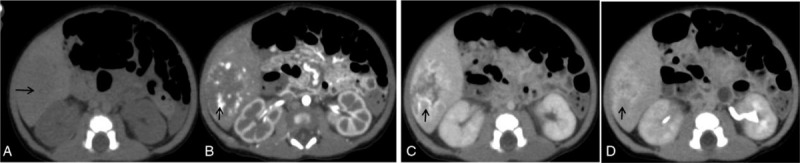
A 2-day-old female neonate with CHB in liver segment SVI, with a maximum diameter of 4.4 cm. A. Small patchy slightly high-density microhemorrhages (black arrow)in the mass was indistinctly seen on the unenhanced CT; B. There were multiple nodular distinct enhancement (black arrow) in the center and periphery of the mass on the arterial phase. C, D. The enhancement of the mass presented progressive expansion and fusion filling (black arrow) on the portal and delayed phases, and finally achieved nearly complete but inhomogeneous enhancement. CHB = congenital hepatoblastoma, CT = computed tomography.
